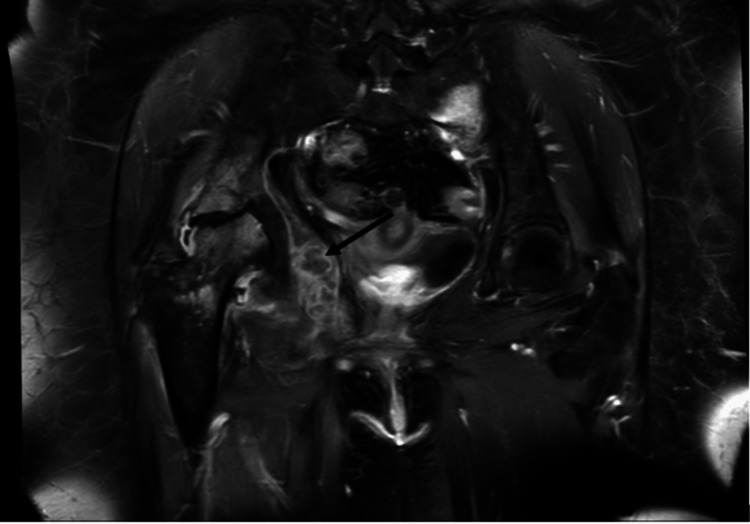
MRI of pelvis showing the peripherally-enhanced fluid collection (arrow) in the right obturator internus muscle measuring 3.7 x 2.1 x 3.9 cm, which may represent an abscess
